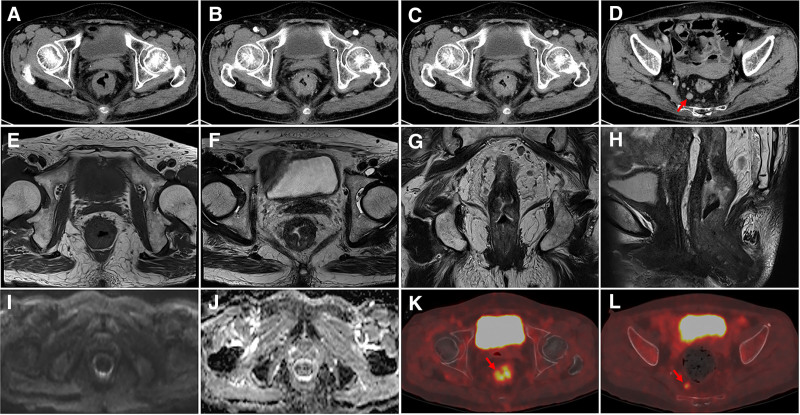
(A–D) The CT images of the patient. Enlarged lymph nodes surrounding the lesions (red arrow). (E–J) The MRI images of the patient. (K, L) The PET-CT images of the patient. the rectal lesion (K, red arrow) and enlarged lymph nodes (L, red arrow). CT = computed tomography, MRI = magnetic resonance imaging, PET-CT = positron emission tomography-computed tomography.
